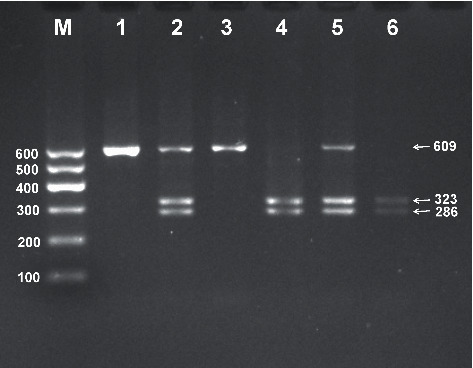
Genotyping of the PLTP rs4810479 SNP. Lane M, 100–600 bp marker ladder; lanes 1 and 3, TT genotype (609 bp); lanes 4 and 6, CC genotype (323 and 286 bp); lanes 2 and 5, CT genotype (609, 323, and 286 bp).
